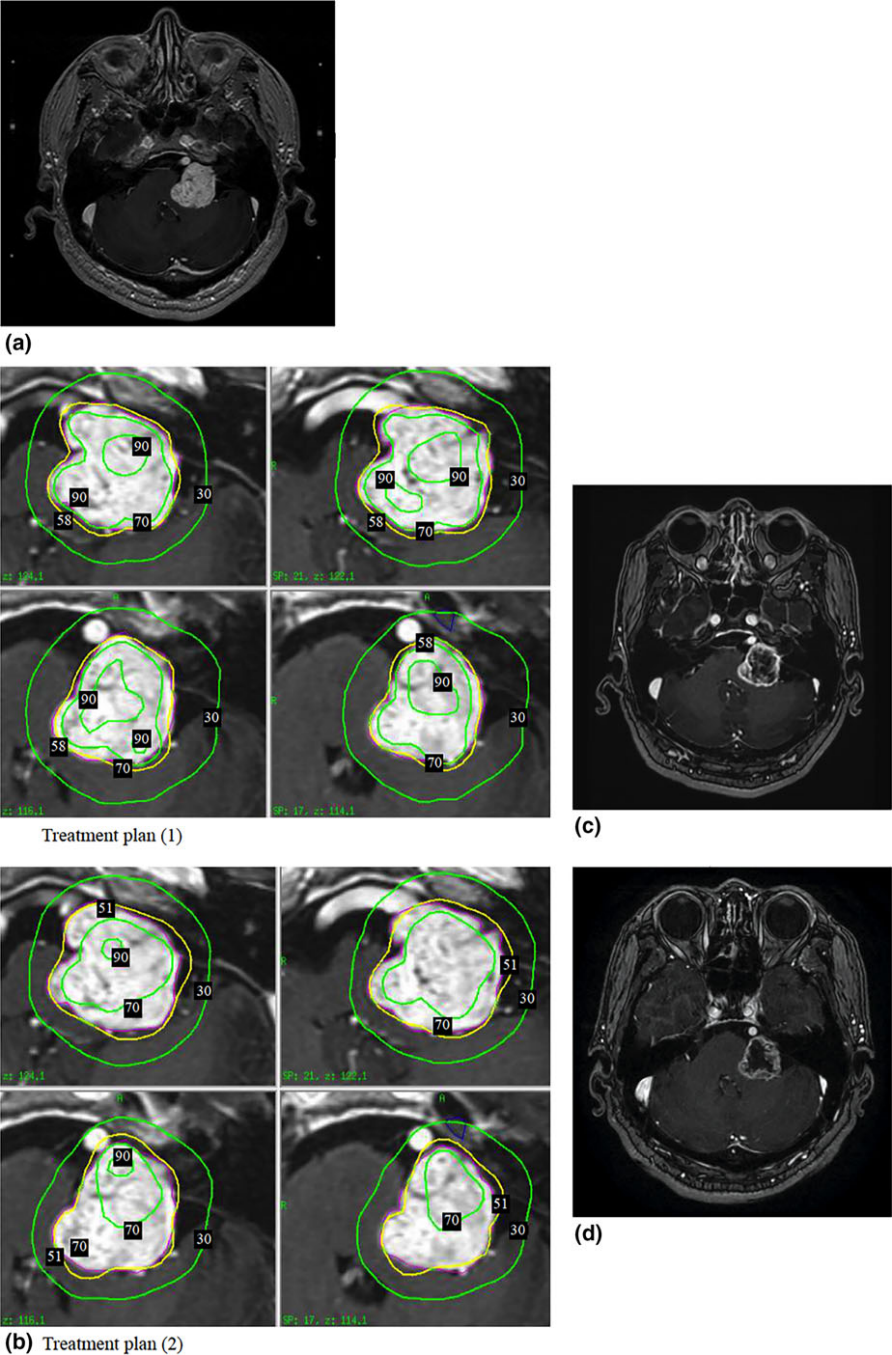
(a) A left acoustic neuroma with a volume of 11.5 cm^3^ was treated by GKRS. (b) Comparison of two treatment plans for this tumor, plan (1) with the normalization effect reduction and plan (2) without the normalization effect reduction. The tumor was treated by using treatment plan (1). (c) Follow‐up MRI of the same tumor at 6 months after treatment revealed an obvious loss of contrast enhancement with shrinkage of the tumor volume from 11.5 to 8.7 cm^3^. No adverse radiation reactions were observed. (d) Follow‐up MRI 12 months after treatment showed that the tumor volume further regressed to 8.3 cm^3^.
